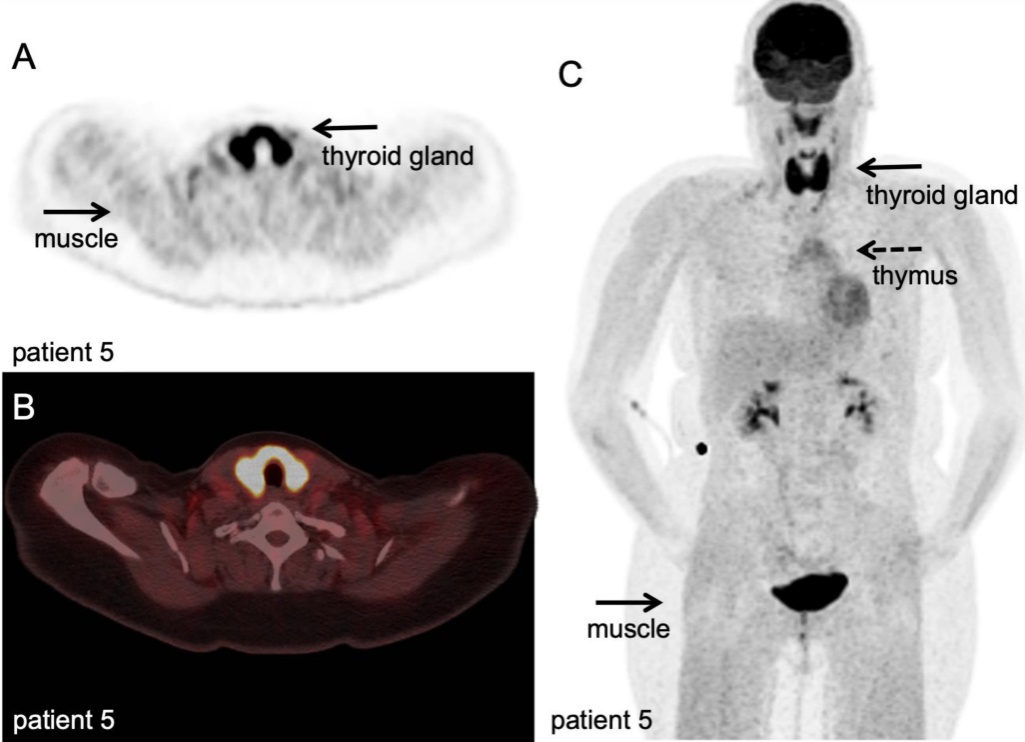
[^18^F]FDG PET/CT example of immune related adverse events. Hodgkin lymphoma patient under PD-1 targeting immunotherapy presenting with myalgia and muscle weakness, scanned at 60 minutes after IV injection of 298 MBq. A-B: transversal PET and fused PET/CT images; diffuse high [^18^F]FDG uptake in the thyroid gland, indicative of thyroiditis, and diffuse markedly increased [^18^F]FDG uptake in all muscles, matching the clinical symptoms of myositis. C: Maximum intensity projection; in addition to thyroiditis and myositis as immune-related adverse events, diffuse increased [^18^F]FDG uptake in the thymus. *(unpublished results)*
